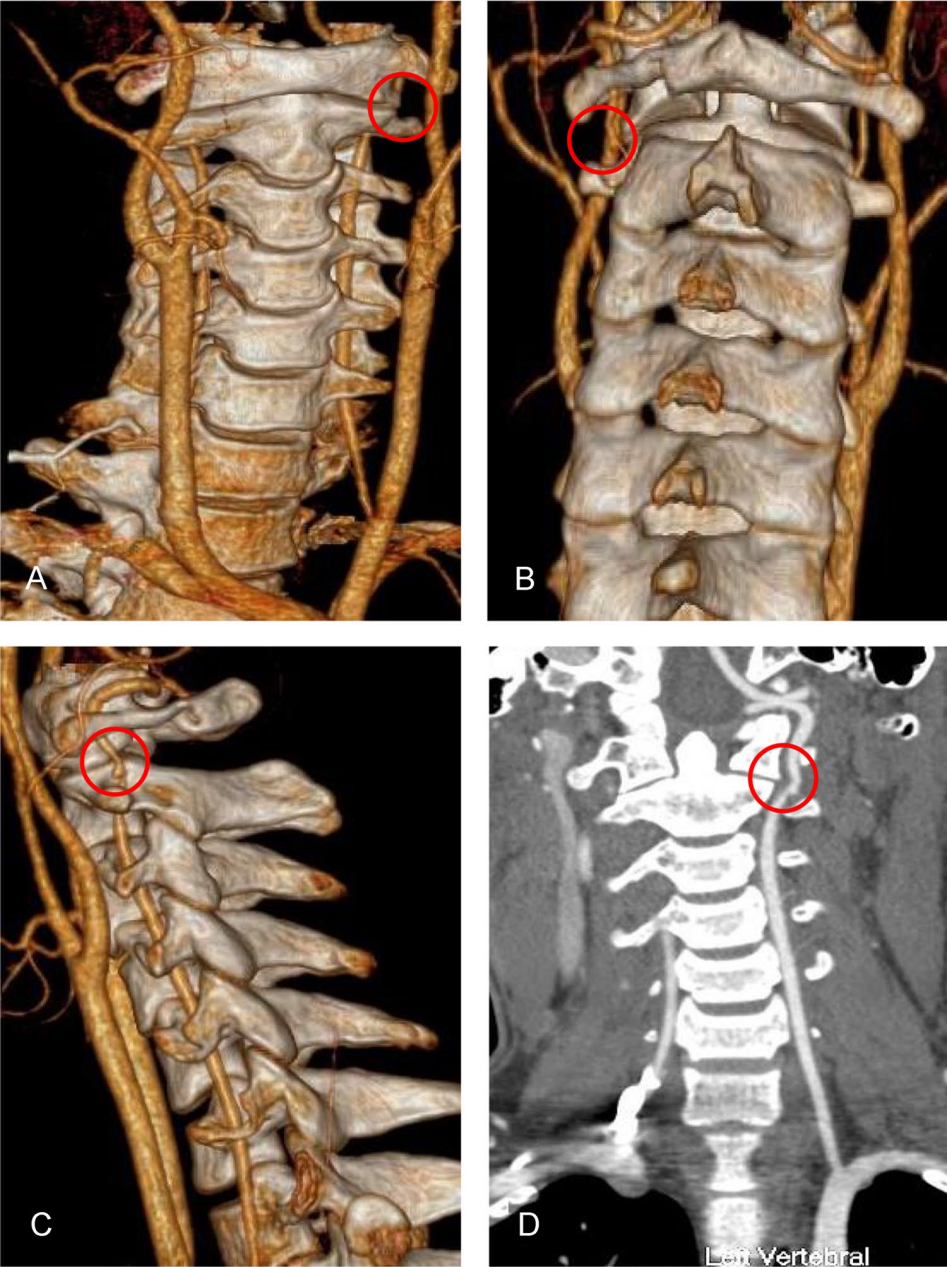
Postoperative 3D-computed tomography and Computed tomography angiography. Postoperative 3D-computed tomography (A, B, C) of the patient’s neck confirmed resection of the osteophyte and a part of C2 transverse foramen unroofing for the left vertebral artery decompression (red circle). Postoperative Computed tomography angiography (D) of the patient’s neck showed the left vertebral artery was fully decompressed and the C1 left osteophyte had been removed (red circle) (Color figure online)
